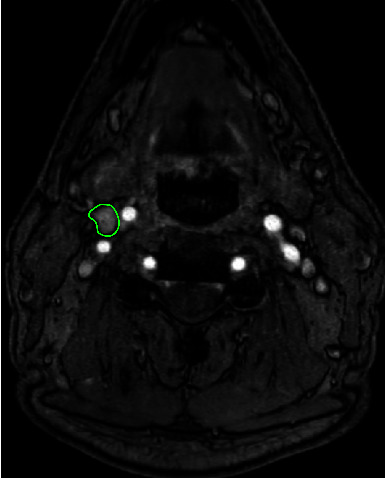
3D TOF with gadolinium MRI showing residual carotic body tumor on the right side (green outline).
